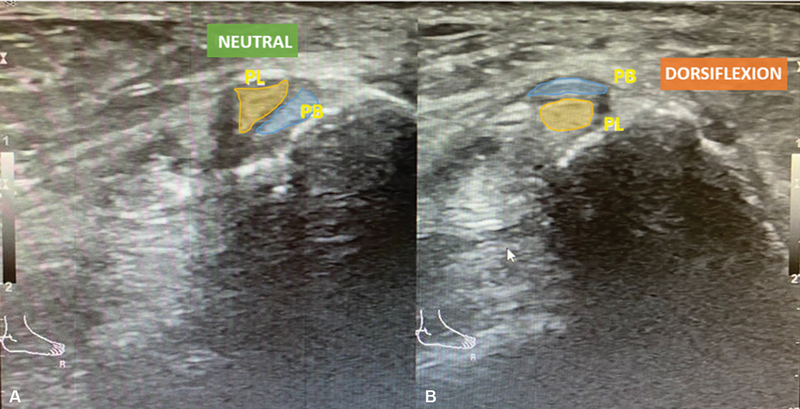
Diagnostic dynamic ultrasound of the lateral ankle showing normal retromalleolar position of peroneal tendons at rest (
